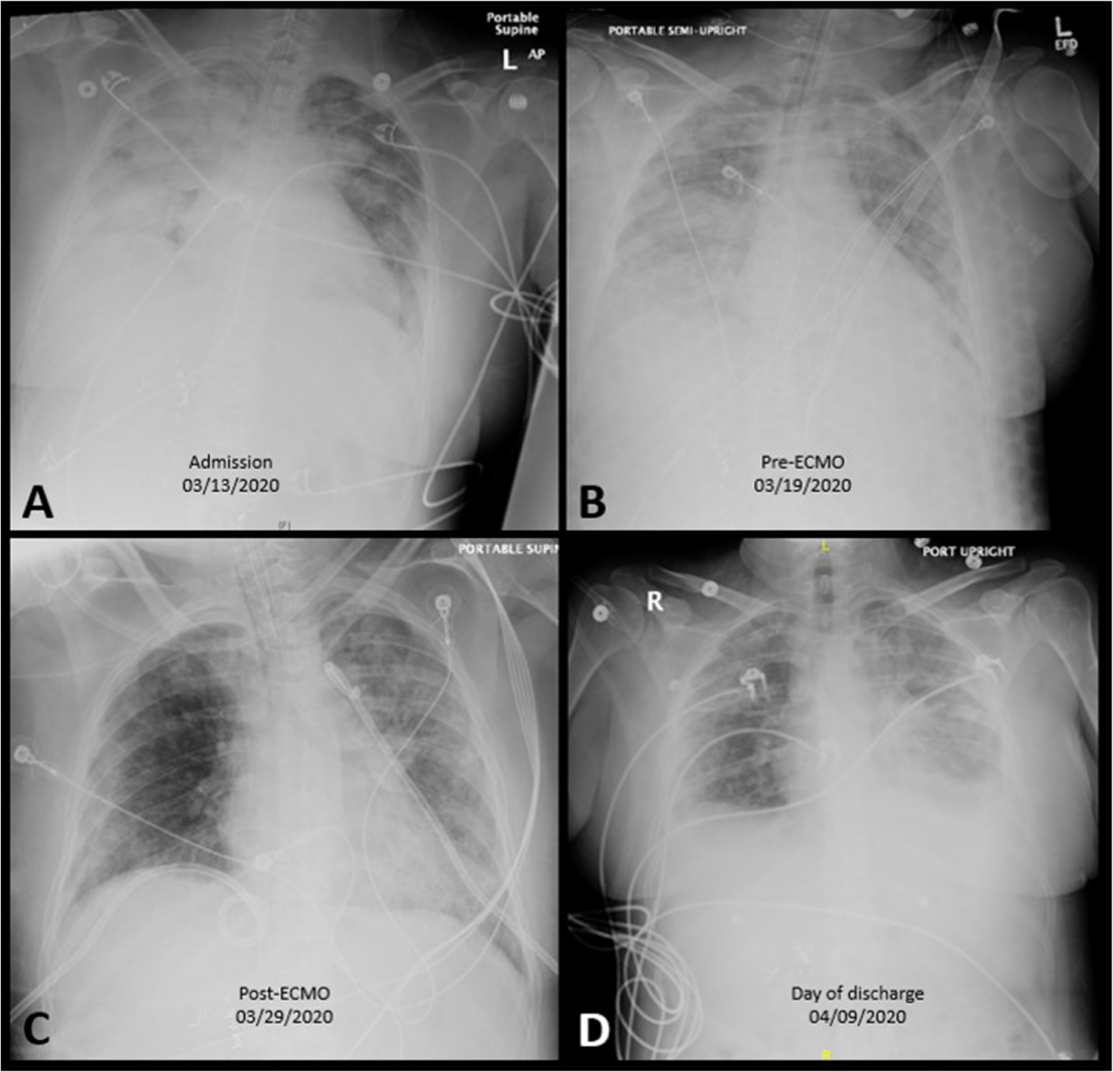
The patient’s chest X-rays on the day of admission (a); day of ECMO cannulation (b); day of ECMO decannulation (c); and day of discharge to rehabilitation (d)
